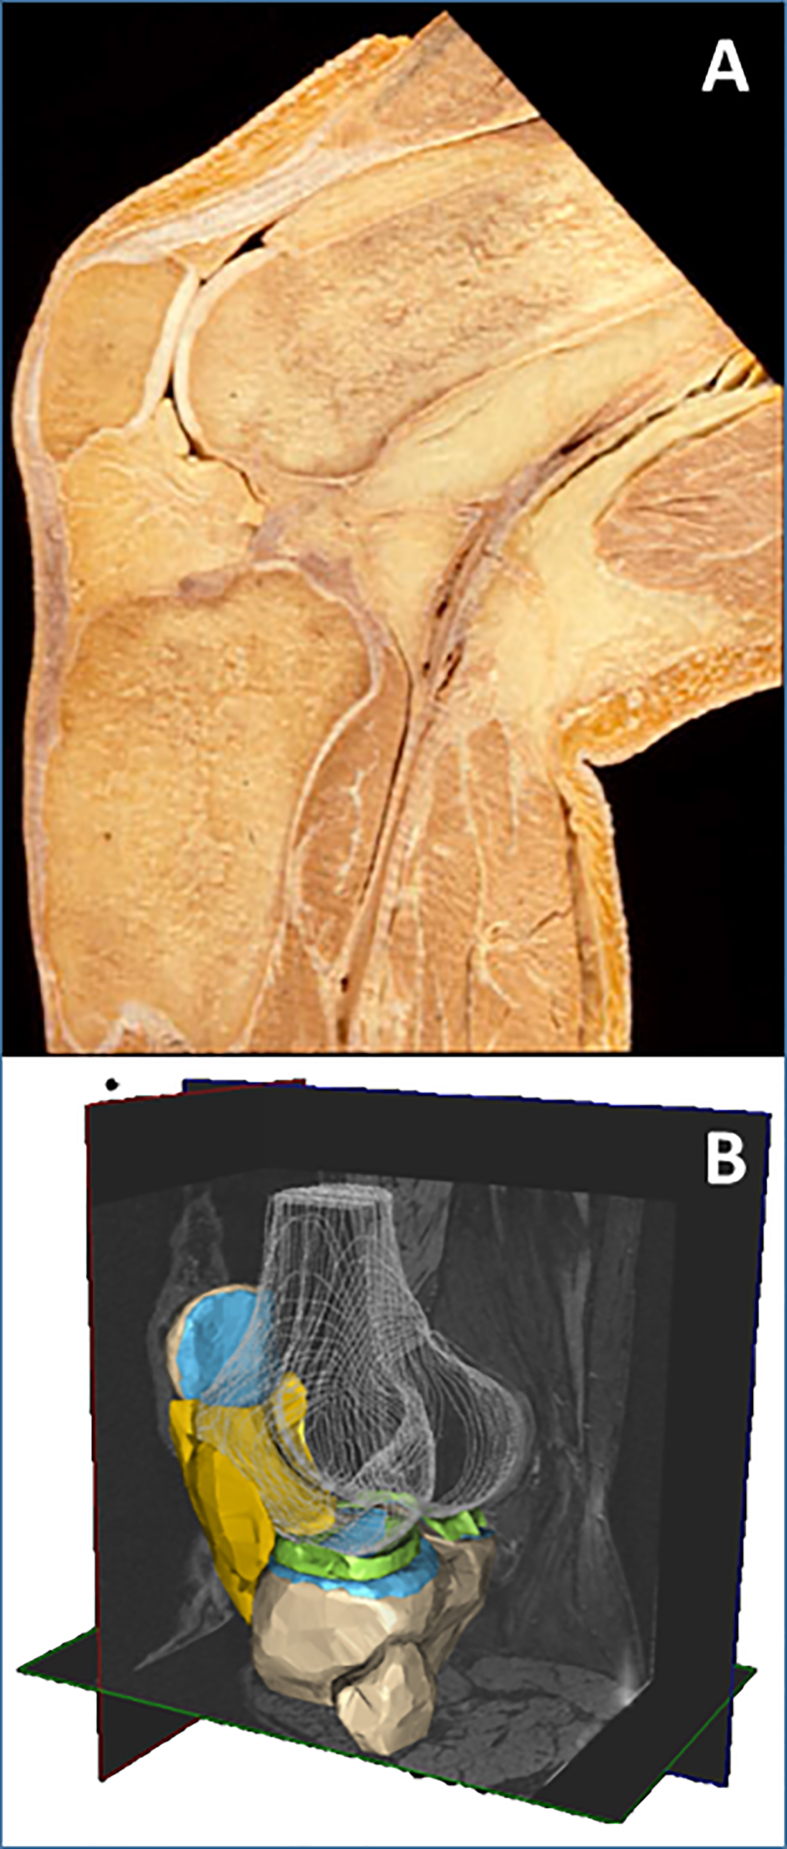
The infra-patellar fat pad (IPFP) or Hoffa: A) Sagittal anatomical section through the knee displaying the IPFP between the patella, distal femur, and proximal tibia; B) 3D reconstruction of (peri-) articular tissues of the knee from MRI showing the IPFP in yellow.
